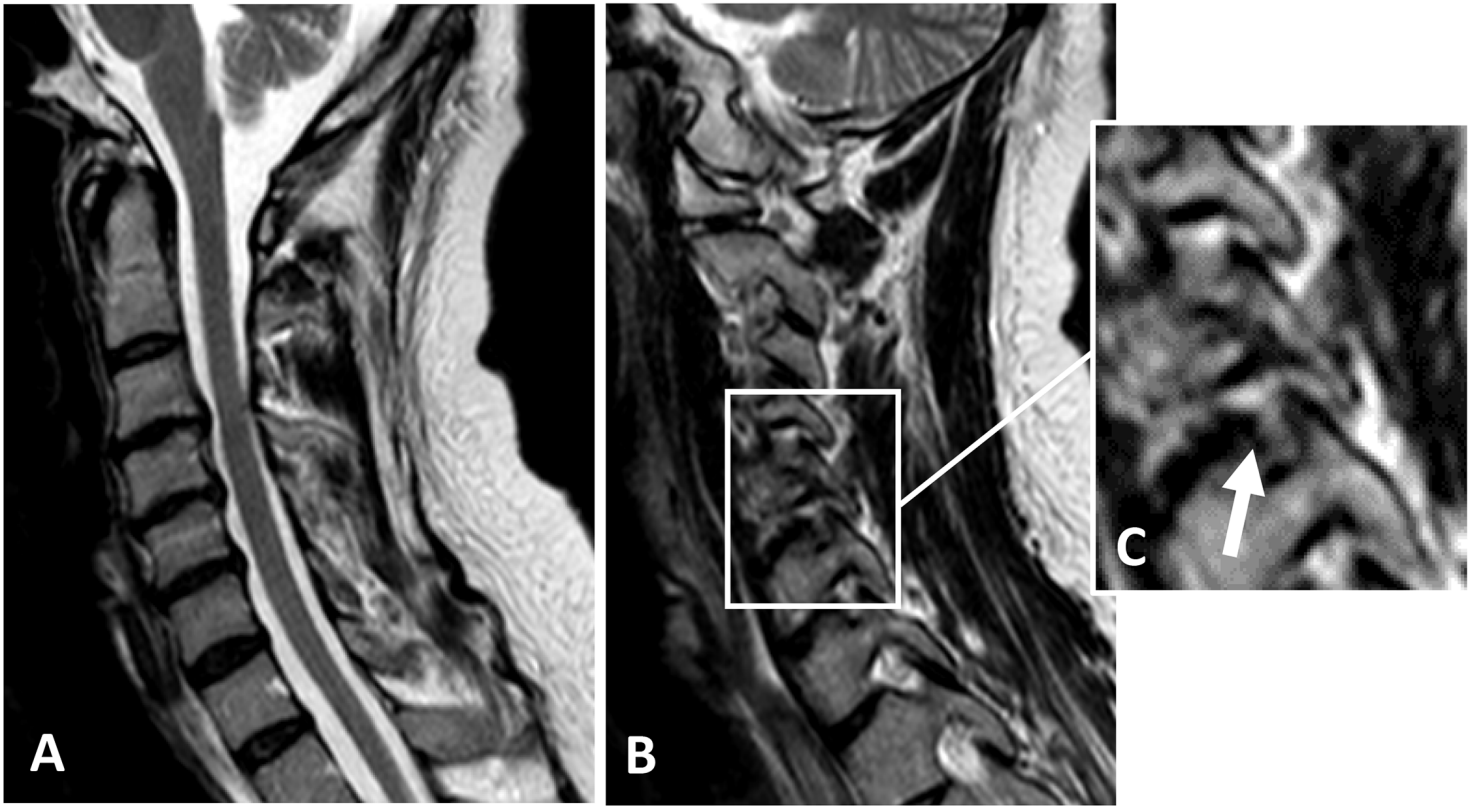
MRI image of cervical spine in TE. MRI of the cervical spine for one of the TE patients where moderate disc degeneration was seen at C3-4, C4-5 and C5-6 level (A). Foraminal narrowing with nerve compromise can be seen here at level C5-6 on the right side (B and enlargement C, with arrow pointing at the disc/bulge/nerve compromise).
